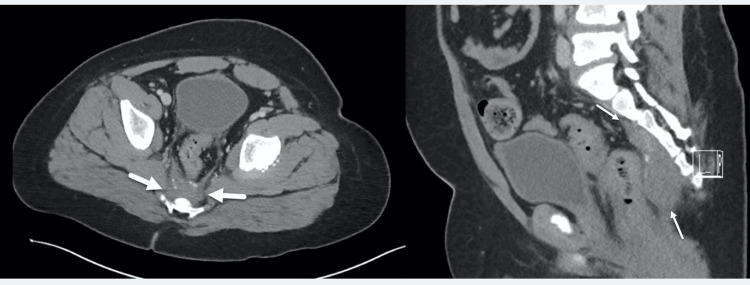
Pelvic CT. Axial (a) and sagittal (b) CT images of the pelvis demonstrate a presacral soft tissue mass (arrows) with high-density calcifications. The abundance of subcutaneous fat density from obesity can also be noted.
